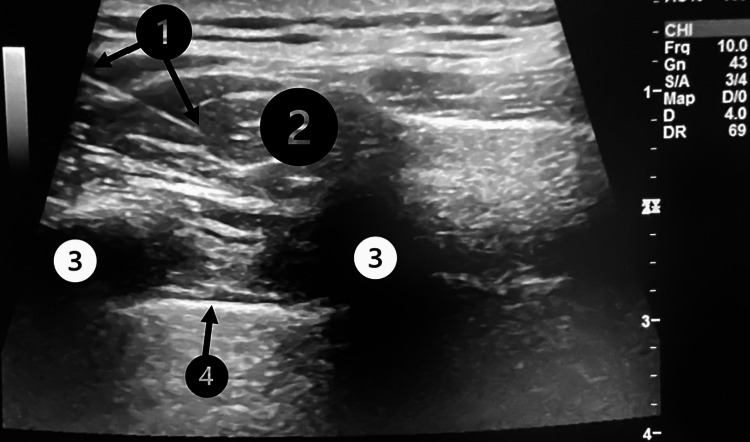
Real ultrasound image obtained during one treatment session The top of the image corresponds to subcutaneous fat. The bottom corresponds to the lung. 1) The acupuncture needle shaft. 2) Target muscle; 3) Ribs; 4) Pleura. Image obtained by Pedro Afonso and edited by Helder Cardoso, with patient consent.
